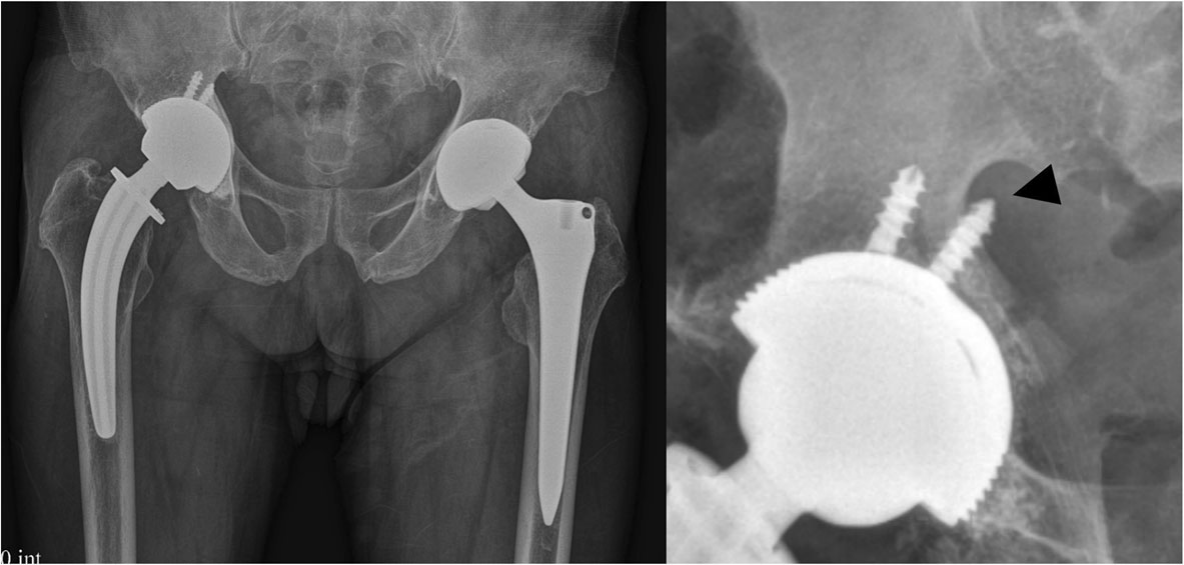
Preoperative hip radiographs (anteroposterior view; Left, iliac oblique view; Right) with protruded dome screw (arrowhead) into sciatic notch
